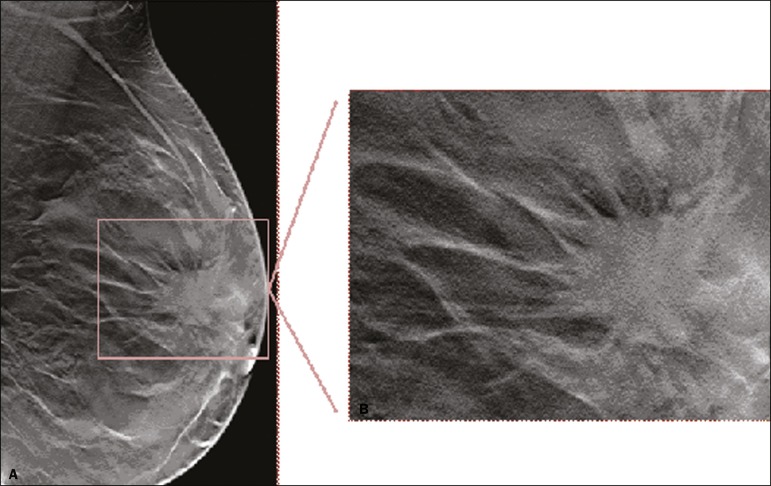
Tomosynthesis, in a mediolateral oblique view, of a 20-year-old woman (case 3)—same case as MRI shown in Figure 7—with GCT of the breast showing a mass with prominent spiculated margins, together with desmoplasia, which is highly suggestive of malignancy. Pathology confirmed the diagnosis of GCT.
